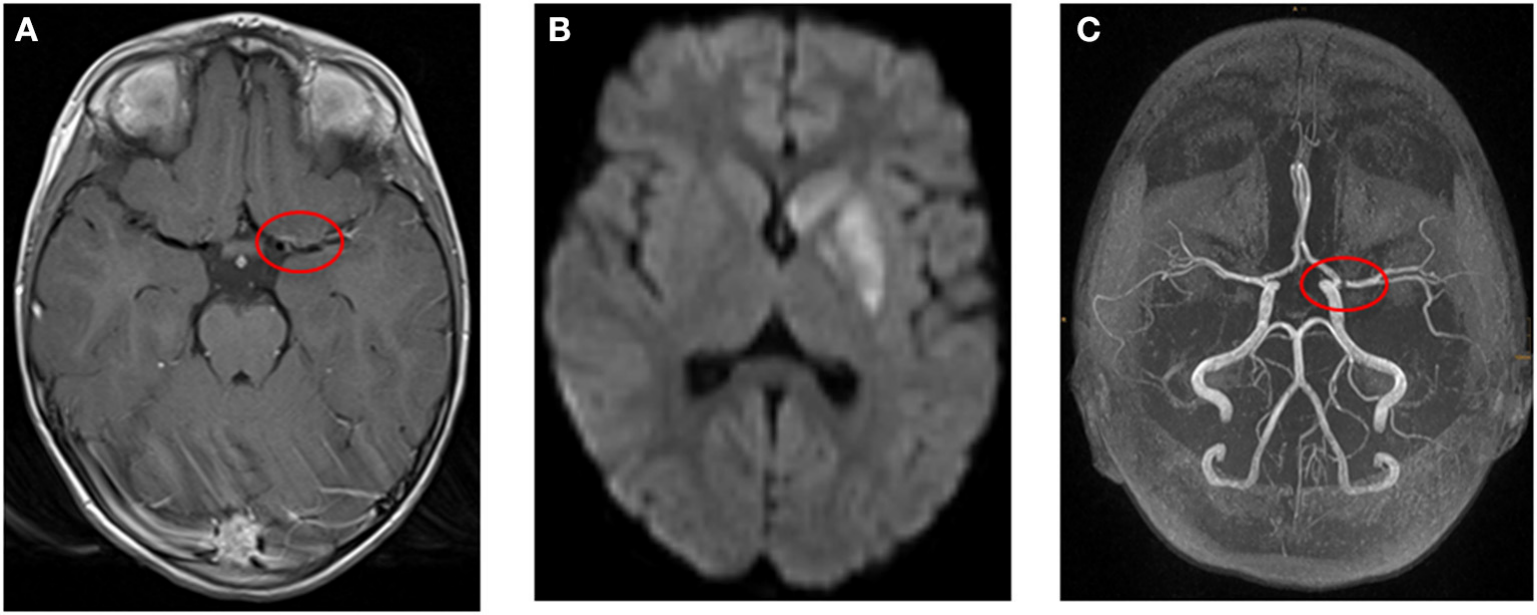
(A–C) Example for a 4-year-old girl with ischemic stroke due to large vessel vasculitis and right-sided hemiparesis and facial palsy. (A) Transversal T1-weighted MR image after contrast shows wall enhancement of the middle cerebral artery (red circle). (B) Transversal diffusion-weighted image (b1000) shows restricted diffusion in the left basal ganglia. (C) Time-of-flight MR angiography demonstrates stenosis of the middle cerebral artery (red circle, left).
